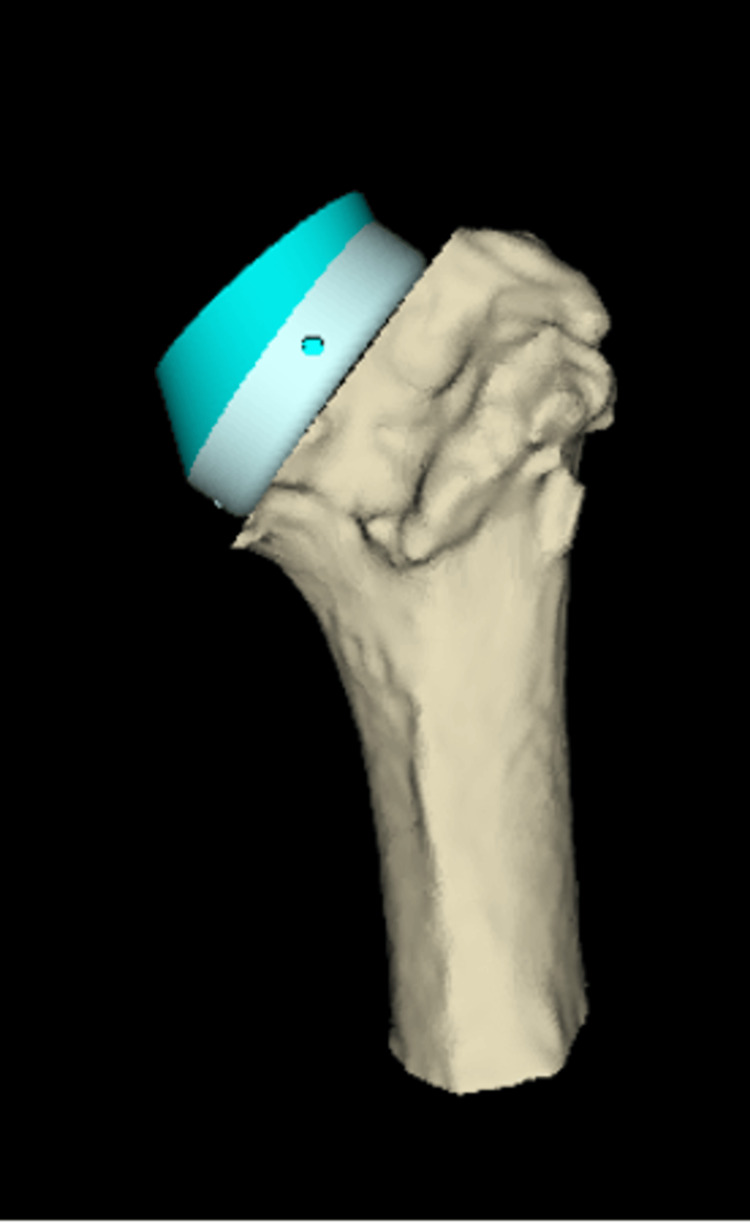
Coronal 3D CT-based planning view demonstrating the humerus with a high-offset tray and +6 mm liner. The configuration demonstrates restoration of humeral length and lateralisation, contributing to improved deltoid tensioning and joint stability.
